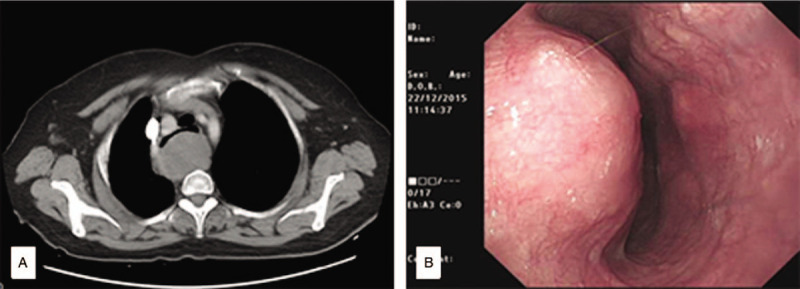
The preoperative radiologic findings. Computed tomography (CT) scan (A) and endoscopy (B) showing the tumor. (A) A CT scan showing a large mass (61 × 46 × 60 mm) in the upper third of the esophagus. (B) Esophagogastroduodenoscopy showed a smooth elevated lesion located 24 to 30 cm from the incisor teeth, the mucosa was intact.
